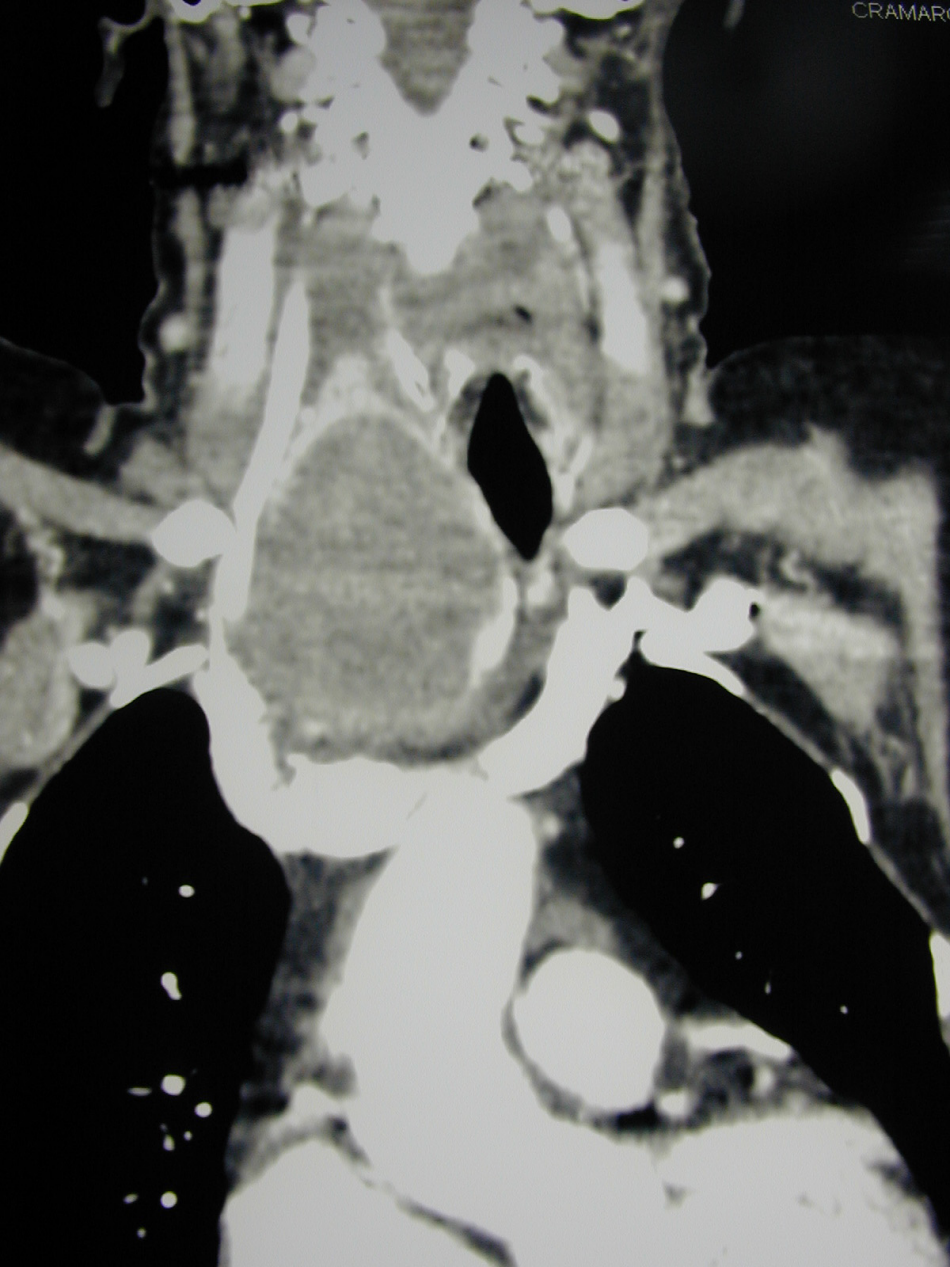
Contrast enhanced CT scan, coronal reconstructed image. The right lobe of the thyroid gland shows a voluminous mass compressing and dislocating trachea, and extending into the upper mediastinum.
